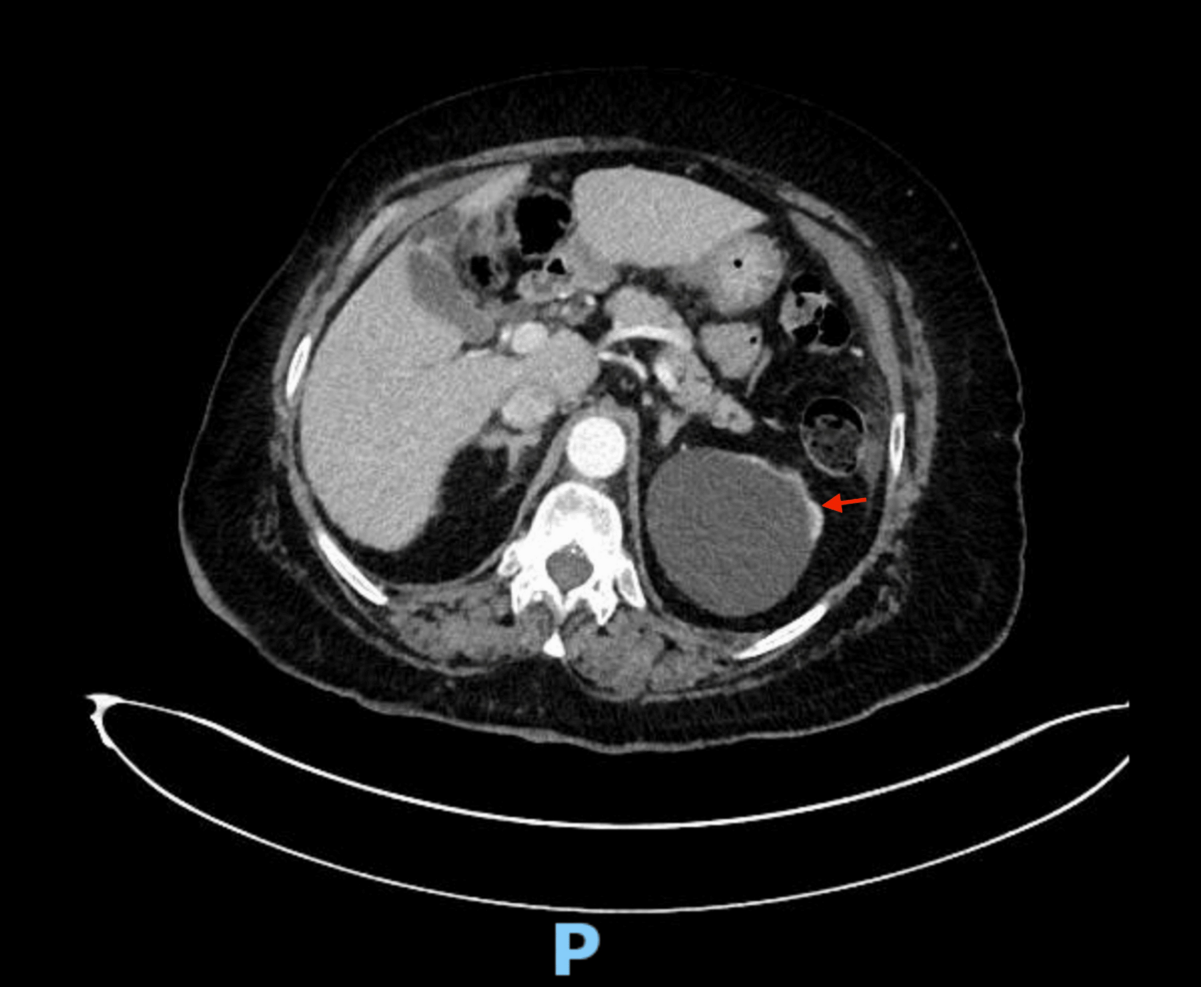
CT abdomen revealing a lower left quadrant 7.5 x 8 cm soft tissue mass. Red arrow indicates the mass.
